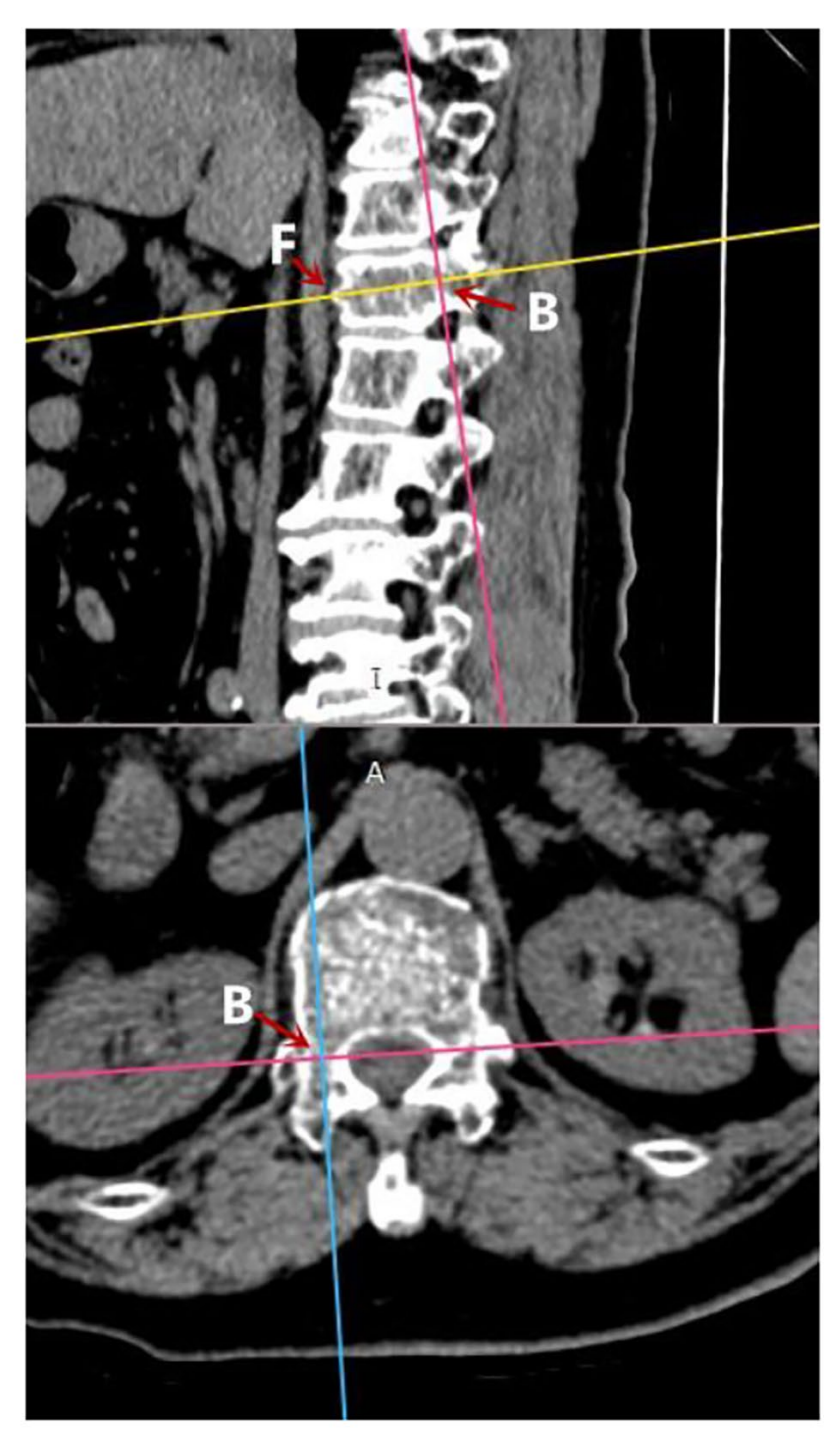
On 3D-CT images of the fractured vertebra, the puncture-side pedicle’s center is designated as point B, and the midpoint of the sagittal anterior edge is point F
